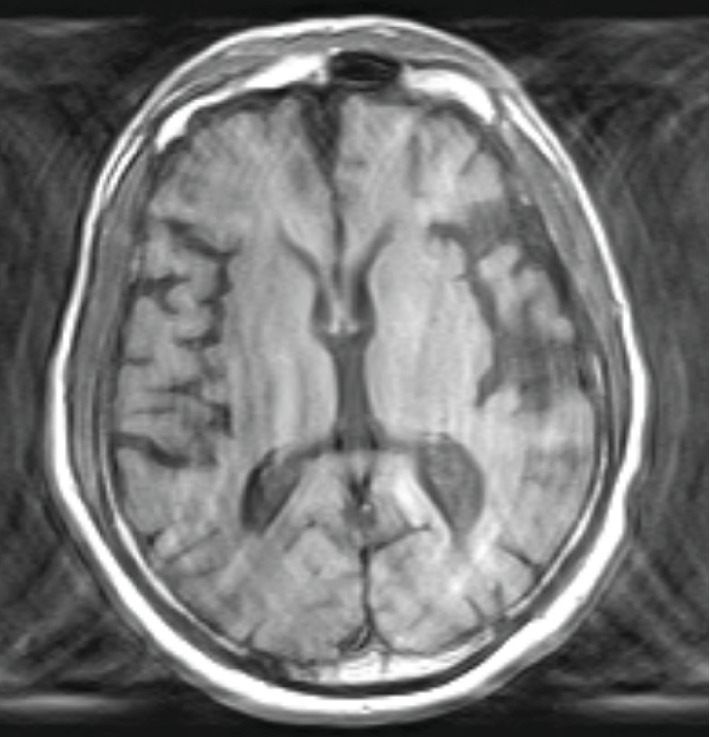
MRI of the brain showing the left parietal, insular, and lacunar infarct and atrophy.
